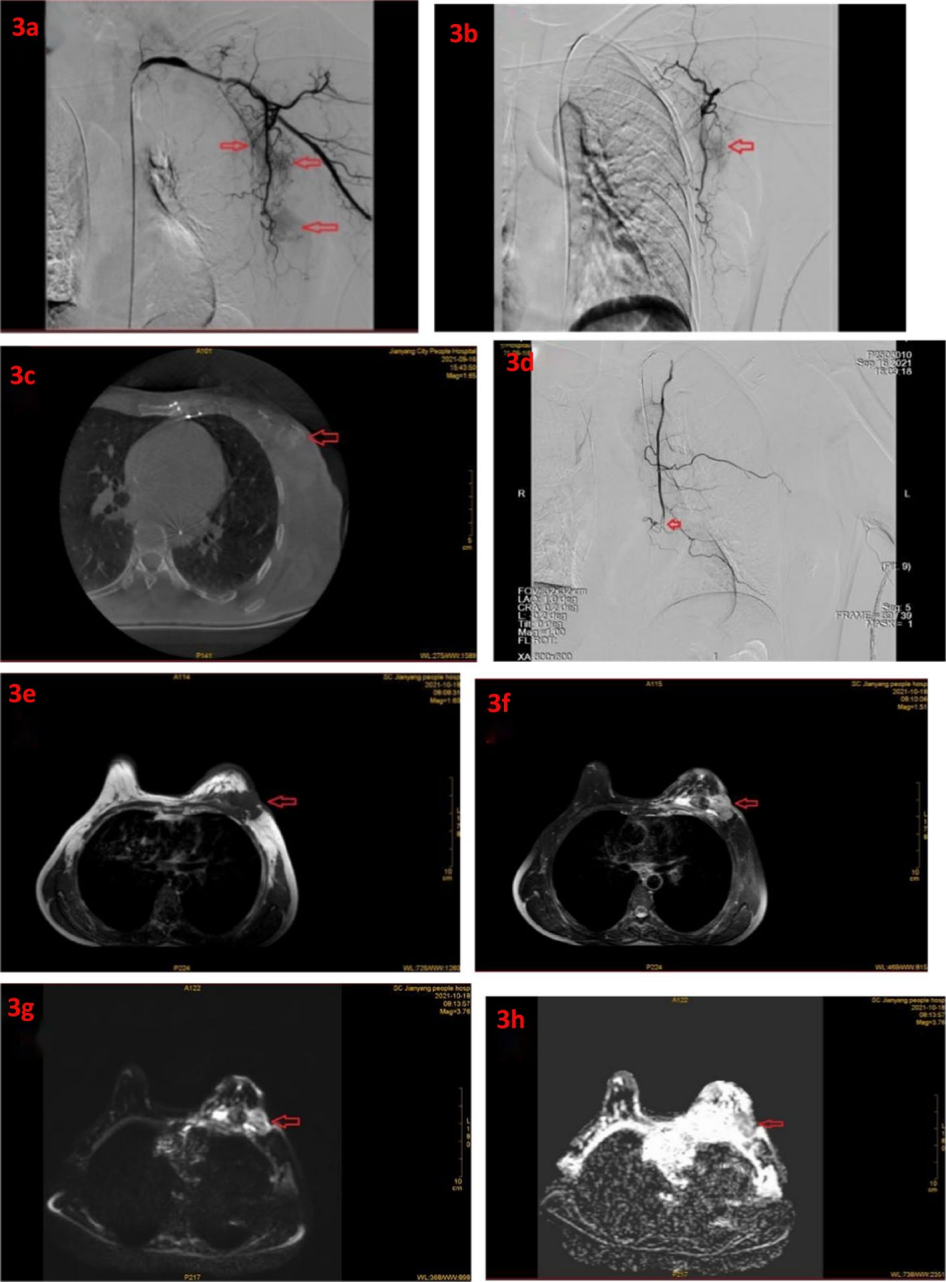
(a), (b) Intraoperative dyeing of breast tumors and axillary lymph nodes with metastasis; (c) Under CBCT, breast tumors are displayed; (d) Post-embolization contrast-enhanced images, with an arrow indicating a metallic coil; (e) T1-weighted imaging (T1WI); (f) T2-weighted imaging (T2WI); (g) Diffusion-weighted imaging(DWI); (h) Apparentdiffusioncoefficient (ADC).
